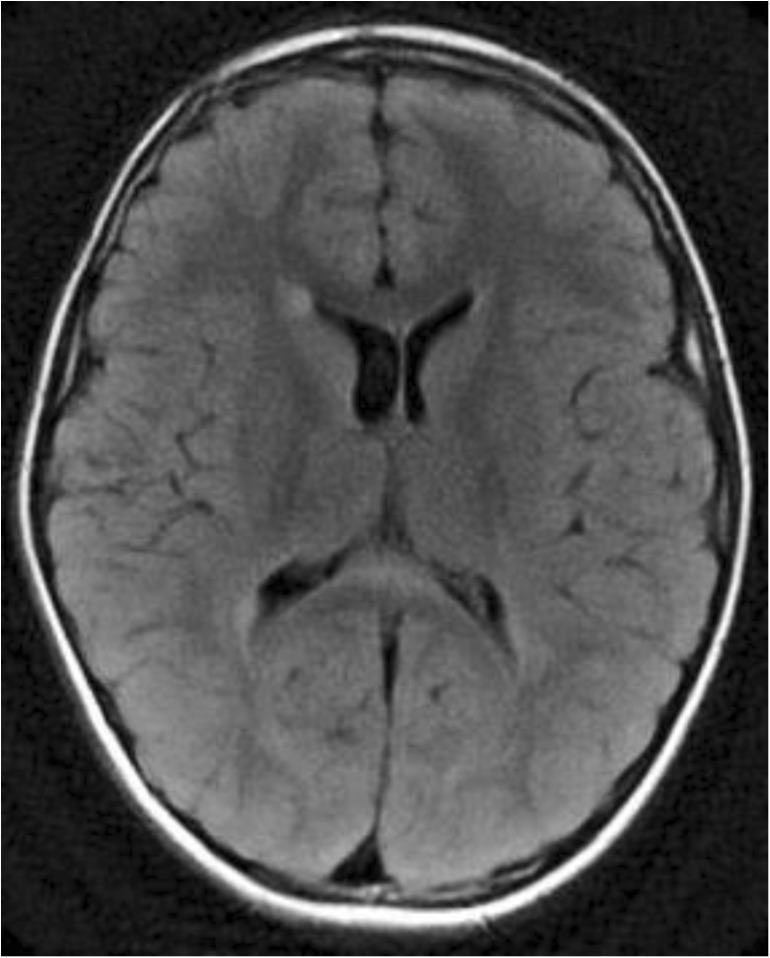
Seven-year-old boy with X-linked adrenoleukodystrophy. Axial FLAIR shows hyperintense area located in the ventral splenium
